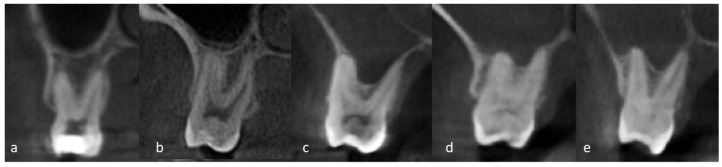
CBCT images showing proximity of the root tips of posterior maxillary teeth (RPMT) to the sinus floor. (a) Type 0: no contact with the cortical borders of the sinus. (b) Type 1: the root tips are in contact with the cortical borders of the sinus with an inferiorly curving sinus floor. (c) Type 2: the root tips are projecting laterally on the sinus cavity, but the apex is outside the sinus boundaries with an inferiorly curving sinus floor. (d) Type 3: the root tips are projecting into the sinus cavity with an inferiorly curving sinus floor. (e) Type 4: a superiorly curving sinus floor enveloping part or all of root tips.
